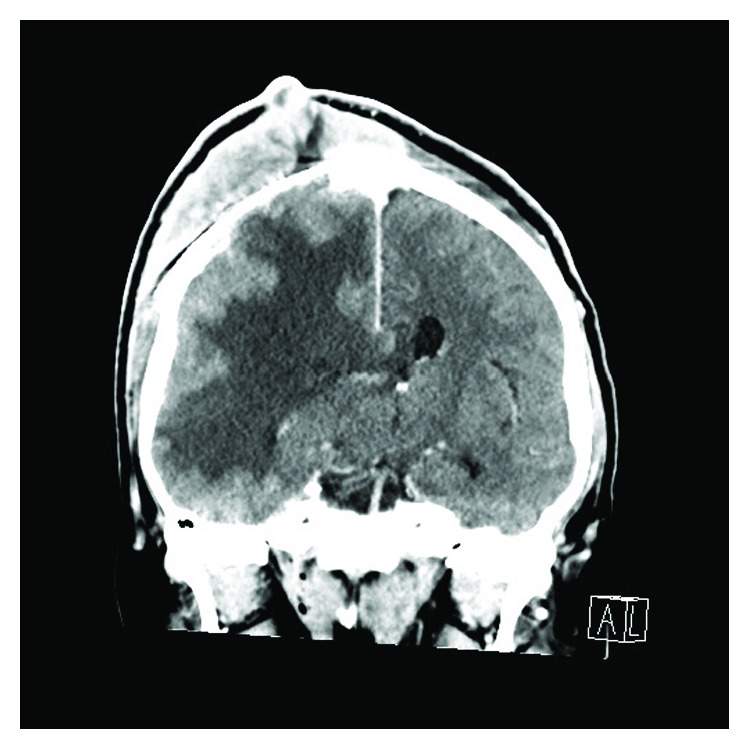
Coronal cut of CT scan of the brain showing tumor masses infiltrating the skull, subcutaneous soft tissue, and skin overlying the previous surgical cavity in which perifocal edema is causing midline shift towards the left hemisphere.
